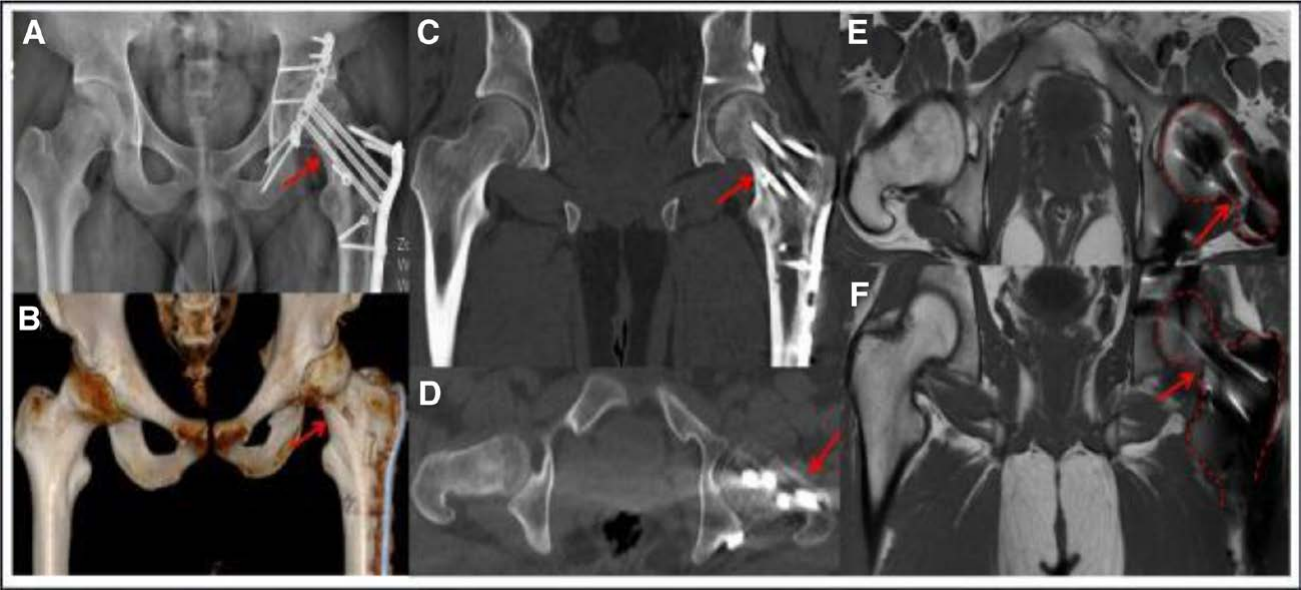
Twenty months after the operation, the patient internal fixation position was consistent with that after the operation. No collapse or necrosis of the femoral head was observed, no hardening of the acetabulum was observed, a large number of callus formed, and the fracture line disappeared. (A) Forward X-ray images of the pelvis; (B) CT 3-dimensional reconstruction of the pelvis; (C) coronal CT scan images of the hip joint; (D) CT scan images of hip joint transversal; (E) MRI images of the transverse hip joint, with red dotted lines representing the femoral head and femoral neck; (F) coronal MRI images and scanning images of the hip joint showed blurred fracture line of the proximal femur, good fracture healing of the femoral neck, and no collapse or necrosis of the femoral head.
